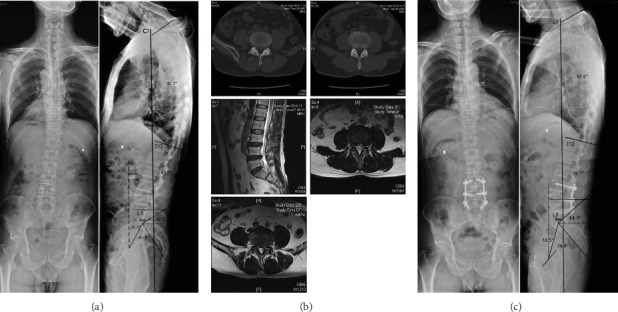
A 60-year-old male with diagnosed with lumbar spinal stenosis and had back pain for 4 years, aggravating pain in both lower limbs for 6 months. (a) Preoperative X-ray showed SVA = 20.4 mm, SS = 32.7, Roussouly type I, with the apex of lordosis at L5 upper edge, GL = 47.7°, PI = 60.8°, PT = 26.6°, SS = 32.7°, GK = 40.7°. (b) Preoperative lumbar CT and MRI showed L3/4, L4/5 segment disc herniation, facet joint hyperplasia and cohesion, and dural compression. (c) Postoperative X-ray showed that SVA = 0 mm, SS = 44.0°, Roussouly type III, the lumbar lordosis vertex was at the L4 midpoint, GL = 55.7°, PI = 58.8°, PT = 16.5°, SS = 44.0°, and GK = 50.0°. SS, GL, and GK increased; SVA and PT decreased; PI unchanged. Lordosis vertex moved up to the L4 midpoint, classification from Roussouly types I to III (standard).
